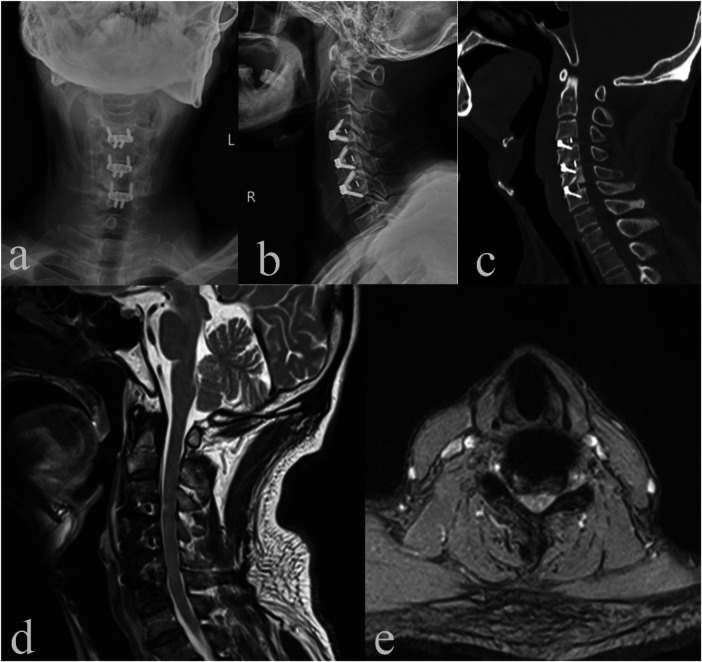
Postoperative cervical spine DR, CT, and MRI. (a) Cervical spine DR anteroposterior view; (b) cervical spine DR lateral view; (c) cervical spine CT sagittal view; (d) cervical spine MRI sagittal view; and (e) cervical spine MRI axial view at C5/6.
